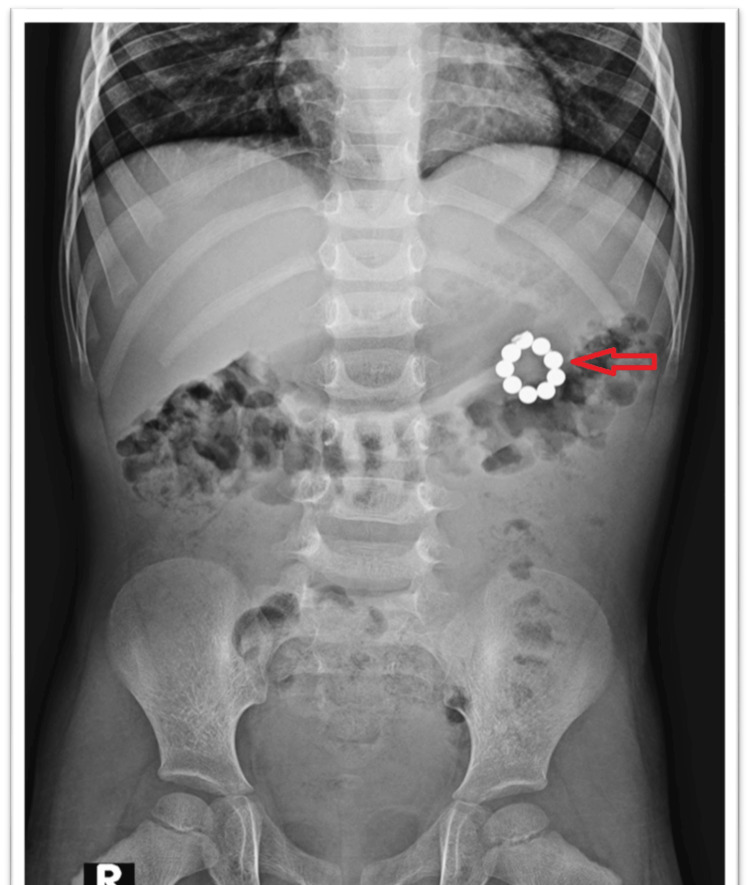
Abdominal X-ray. Plain abdominal radiograph showing multiple magnetic beads arranged in a ring-like pattern (red arrow).
